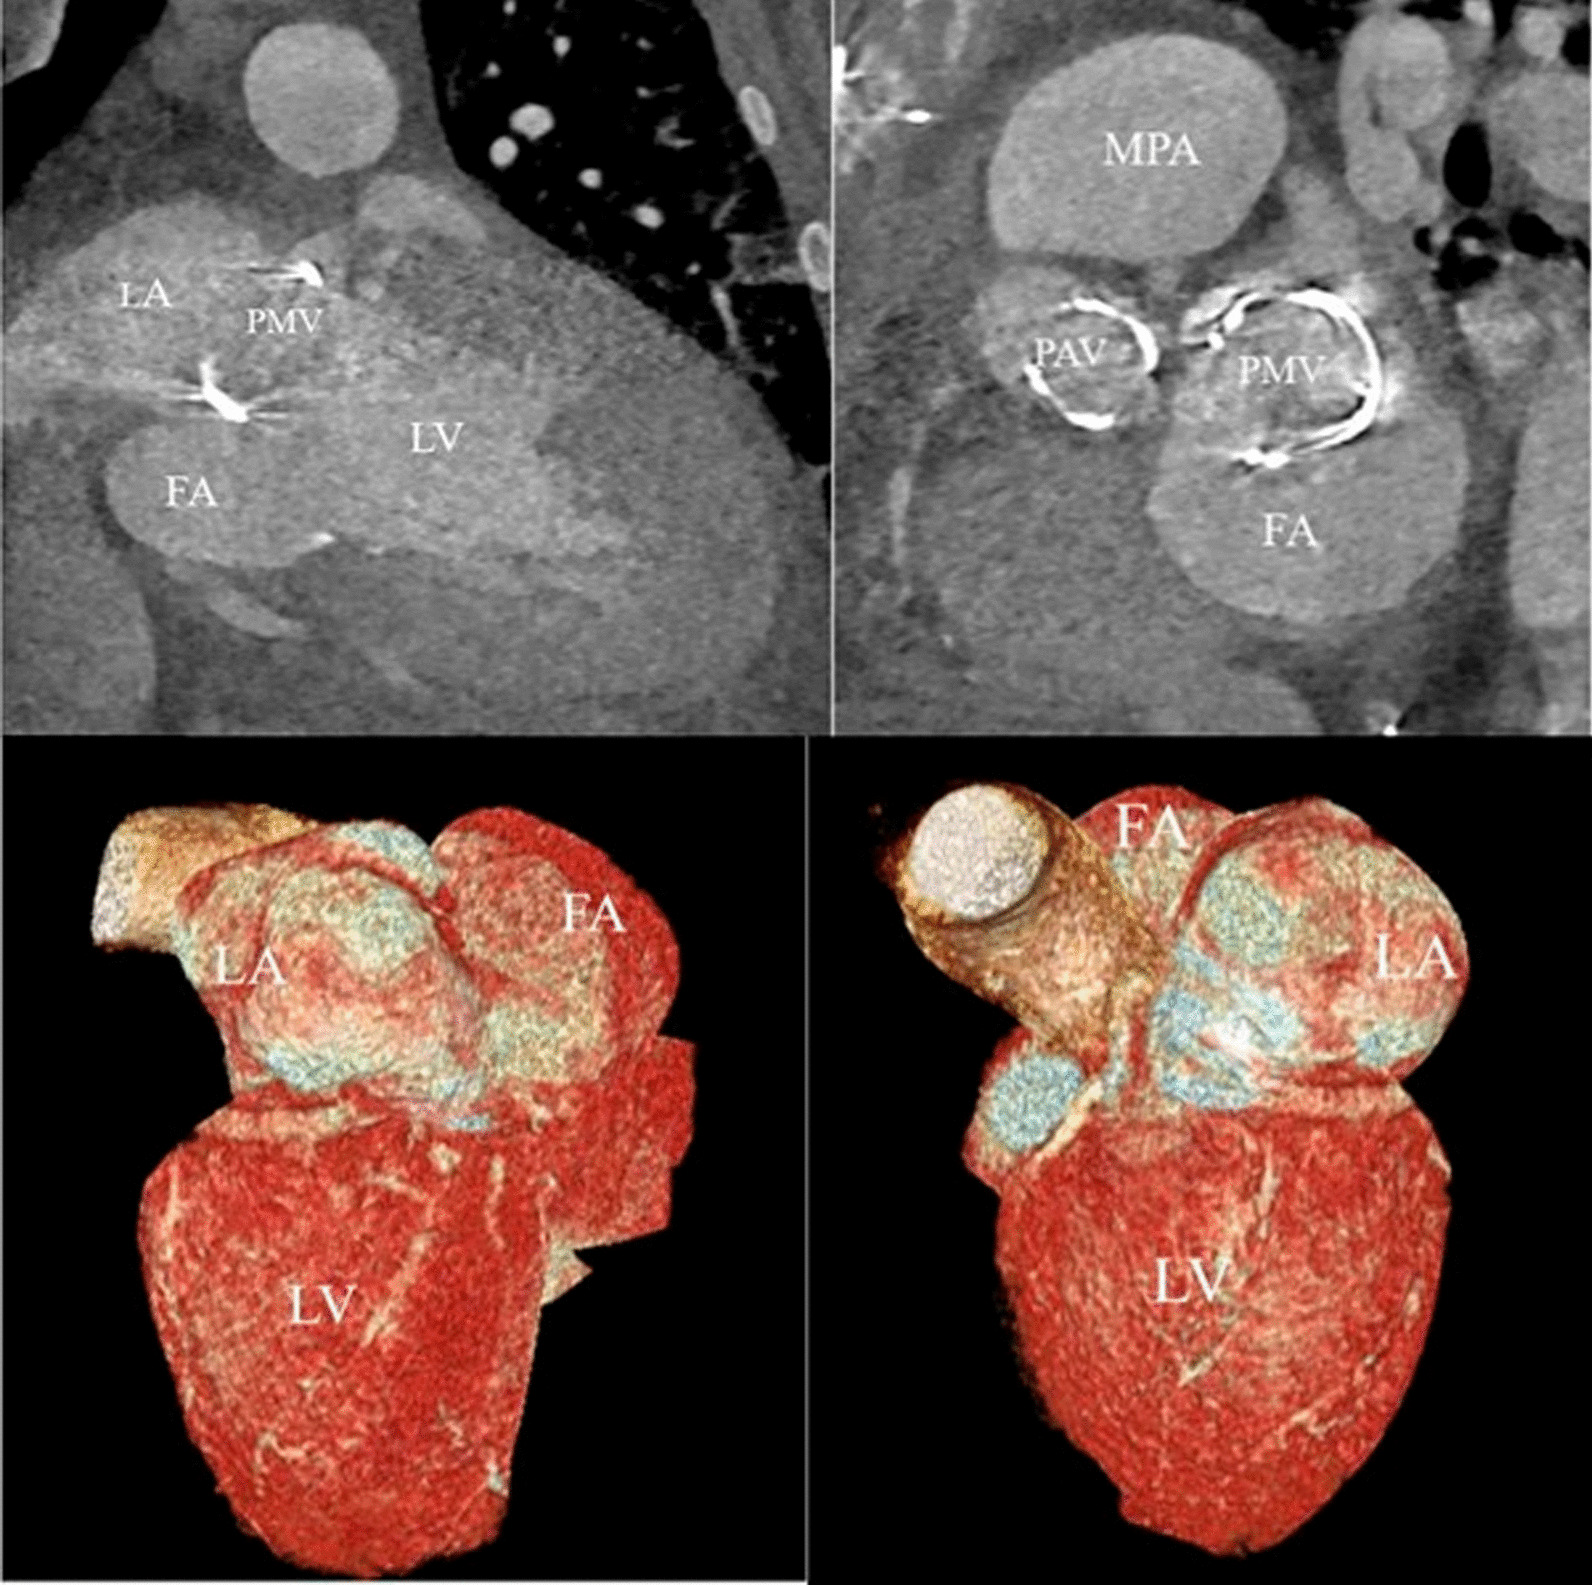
Cardiac CT-scan and 3D-reconstruction. The false aneurysm measures 65 × 40 × 30 mm, behind the prosthetic mitral valve and the left atrium. FA: false aneurysm, LA: left atrium, LV: left ventricle, PAV: prosthetic aortic valve, PMV: prosthetic mitral valve
